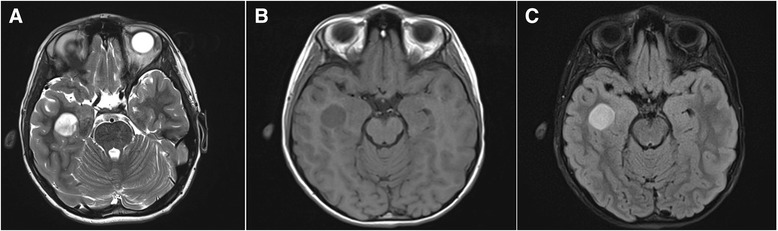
a MRI without contrast, axial section: a T2 hyperintense relatively well defined tumor in the right temporal lobe. b T1 sequence, slightly hyperintense well defined tumor in the right temporal lobe. c FLAIR sequence highlights the tumor; peritumoral edema is not seen
